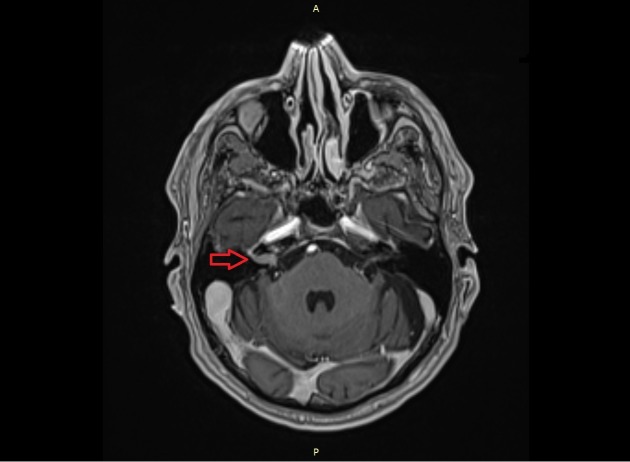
MRI scan. The image shows the rapid progress of the suspected vestibular schwannoma inside the right internal acoustic meatus. MRI: magnetic resonance imaging
